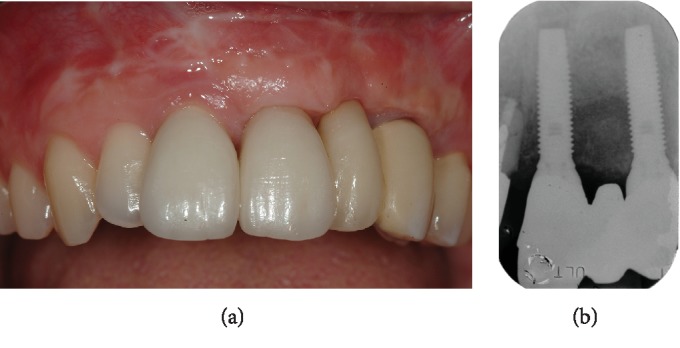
Definitive cemented prosthetic restoration in porcelain-fused-to-metal: (a) clinical view and (b) periapical radiograph.
